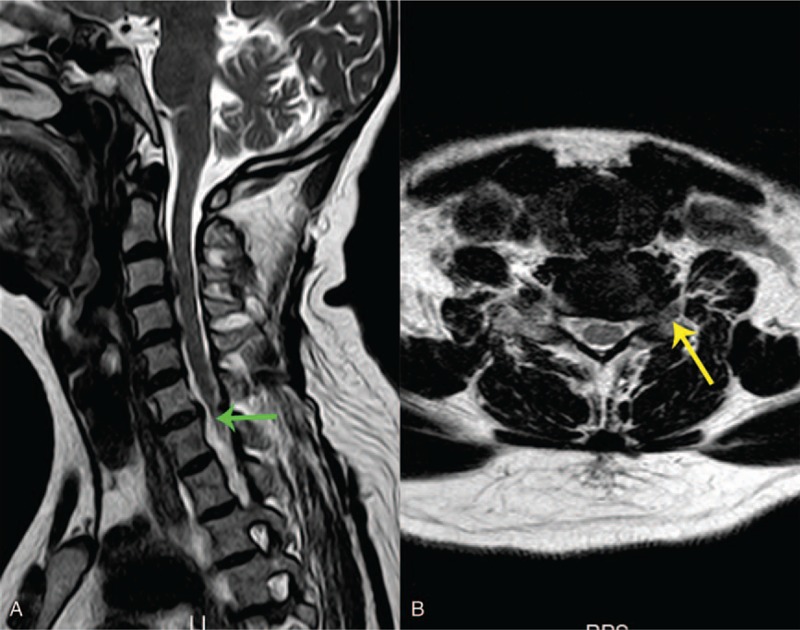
Sagittal (A) and axial (B) of cervical spine magnetic resonance imaging demonstrated that the discs of C3-4 and C6-7 were protruded, and the local dual sac was compressed in an arc. No abnormal signal was found in the spinal cord.
